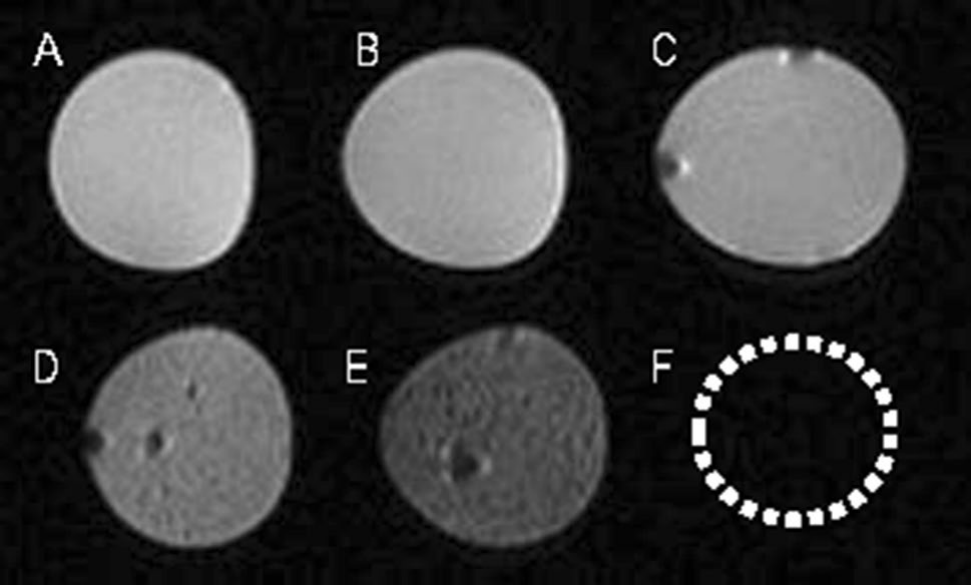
In vitro MR imaging of ADSCs. SPION-labeled ADSCs (D–F) produced a hypointense signal on T2*-weighted MR images compared with distilled water (A), agarose gel (B) and unlabeled ADSCs (C). SPION-labeled ADSCs at a concentration of 1 × 10^6^ (F) showed the greatest hypointense signal than 1 × 10^4^ (D) and 1 × 10^5^ (E). The in-plane resolution was 156 μm × 156 μm. The black spots were actually air bubbles that were not completely removed during the process of embedding cells in 2% agarose.
